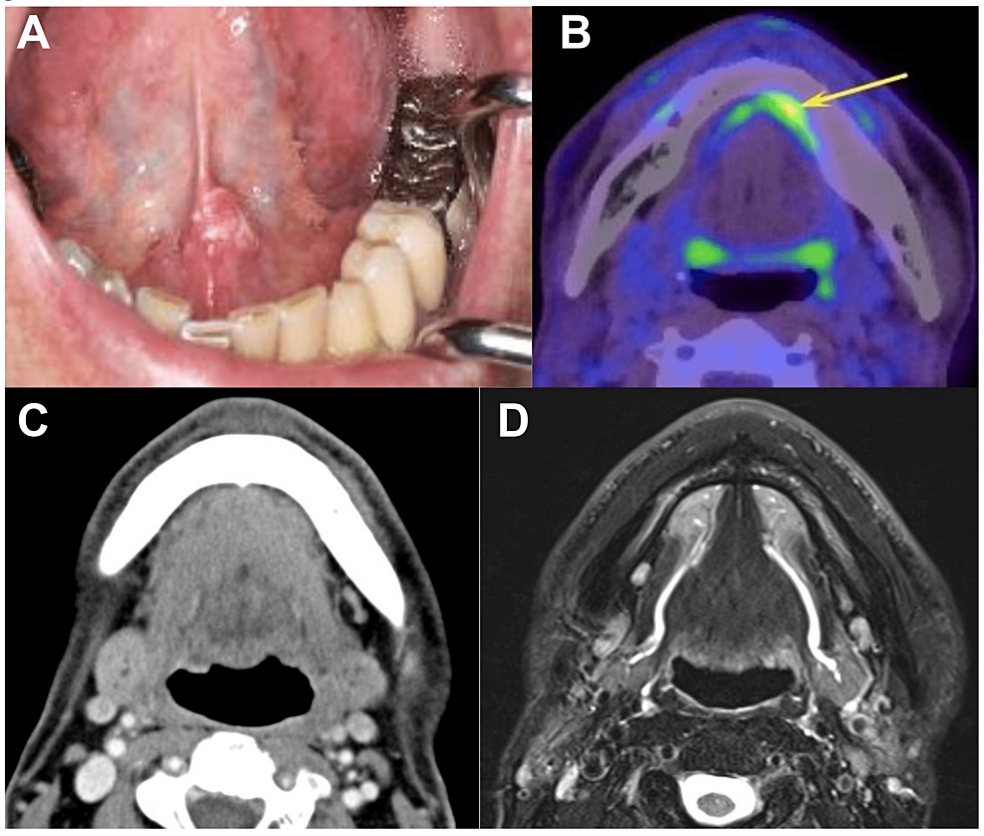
Pretreatment findings. Intraoral photograph: a mass in the left floor of the mouth (A). PET/CT: uptake on the left of the floor of the mouth (yellow arrow) (B). Contrast-enhanced CT: no abnormalities in the left submandibular gland (C). T2WI(FS) MRI: drain from the submandibular glands into the oral cavity via Wharton’s duct (D). PET/CT: Positron emission tomography/computed tomography; CT: computed tomography; T2WI(FS): T2-weighted fat-suppressed; MRI: magnetic resonance imaging
